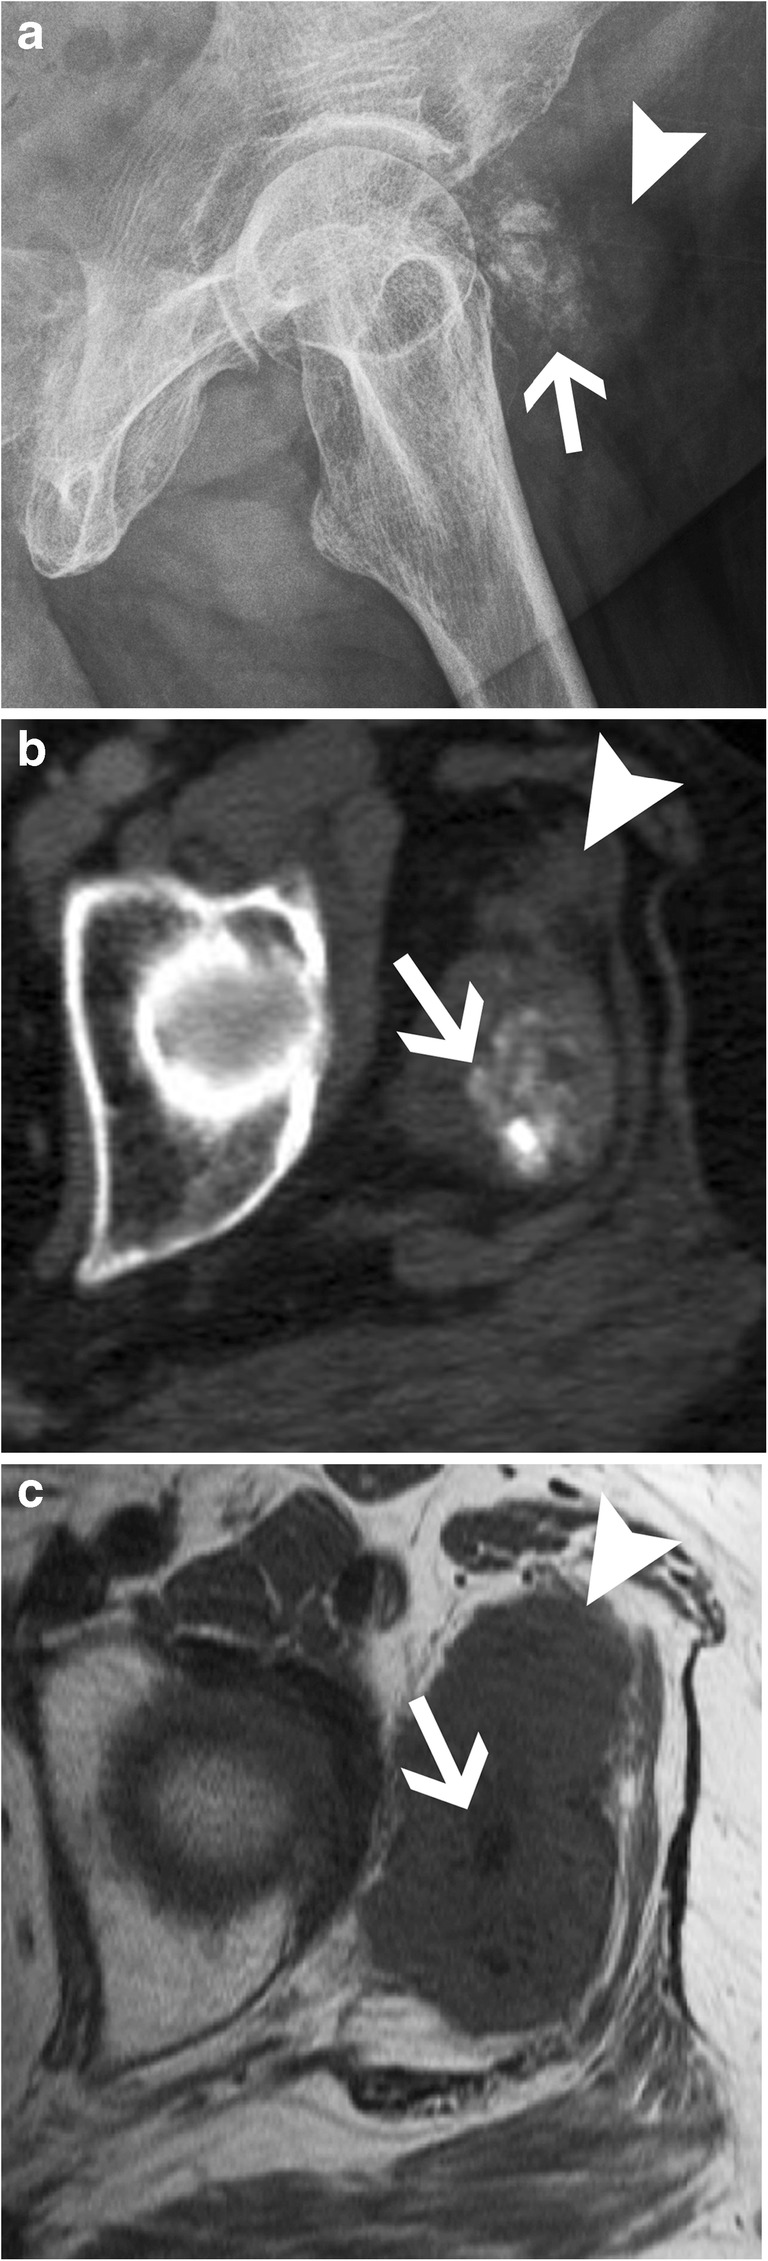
Synovial sarcoma. (a) Frog-leg radiograph, (b) axial CT image and (c) axial T1-weighted image of the left thigh show faint calcifications (arrow in a, b and c) that are located inside a tumour which is better seen on the CT and MRI assessment (arrowhead in a, b and c). Synovial sarcoma was found at pathology
